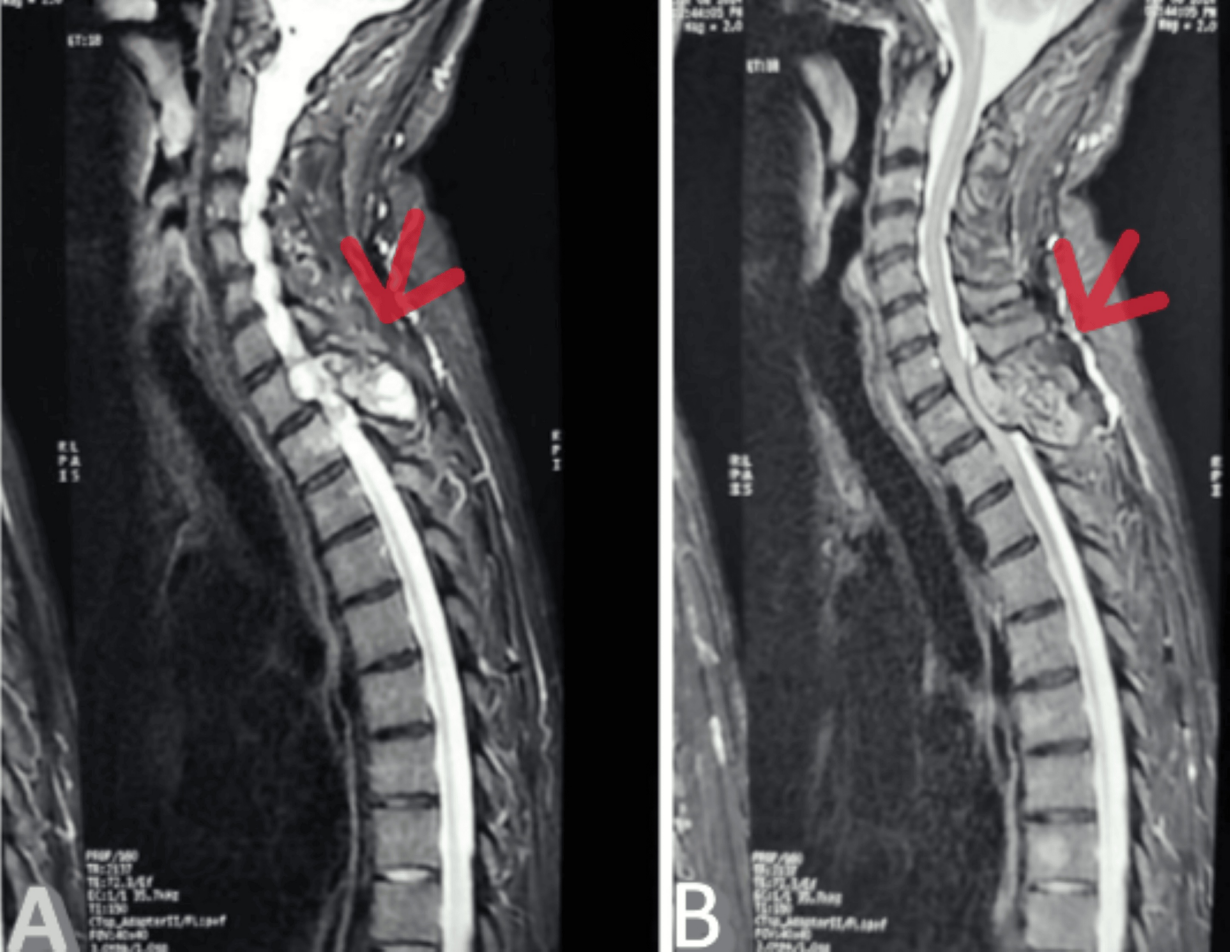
Cervicodorsal MRI in sagittal section showing a lesional process centered on the spinous process of D1 (A) Cervicodorsal MRI in sagittal section T2 sequence showing a lesional process centered on the spinous process of D1, which causes spinal cord compression. (B) Cervicodorsal MRI in sagittal section T1 sequence showing a lesional process centered on the spinous process of D1, which causes spinal cord compression.
